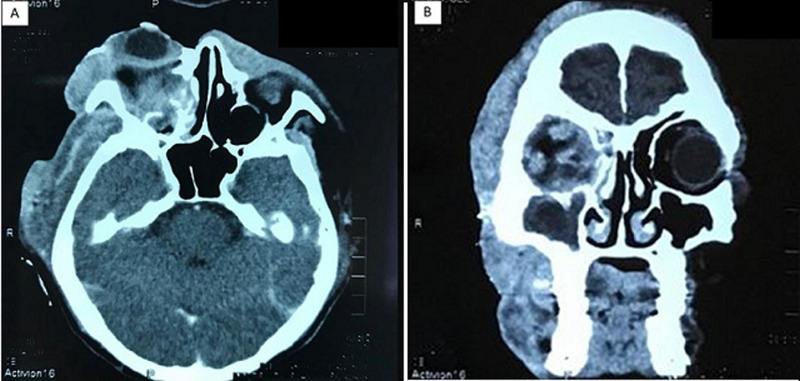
A) head CT scan with contrast, axial slice showed proptosis of the right eye 2.9 cm from interzygomatic line; solid mass was seen in the right retrobulbar, filling intraconal and extraconal area, with heterogenous contrast enhancement; this mass initially raising suspicion of malignancy; B) coronal slices showed thickening of the right extraocular muscles indicating chronic myositis
